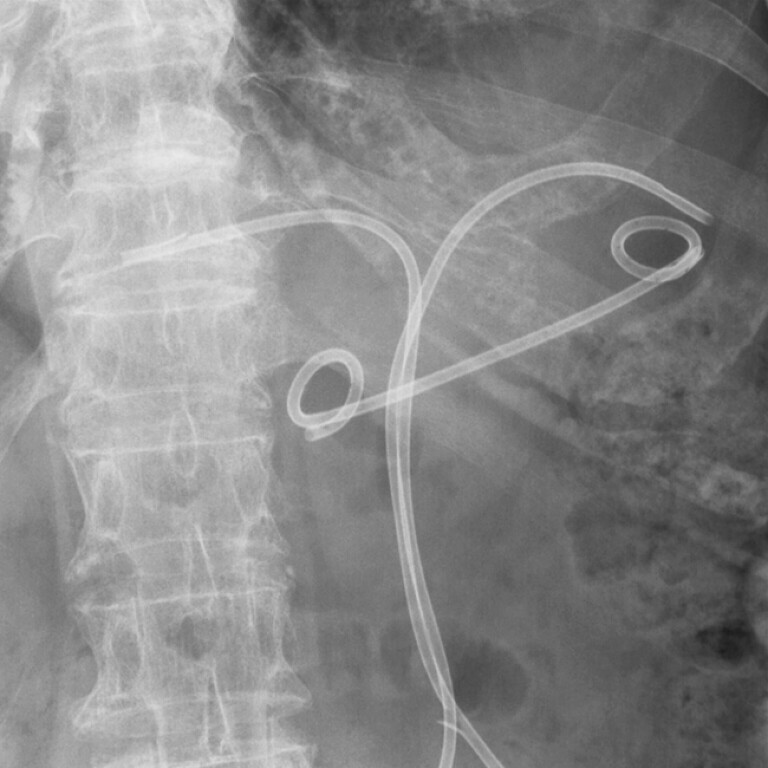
An abdominal radiograph showing the indwelling endoscopic ultrasound-guided gallbladder stent following the removal of the percutaneous drainage catheter.
